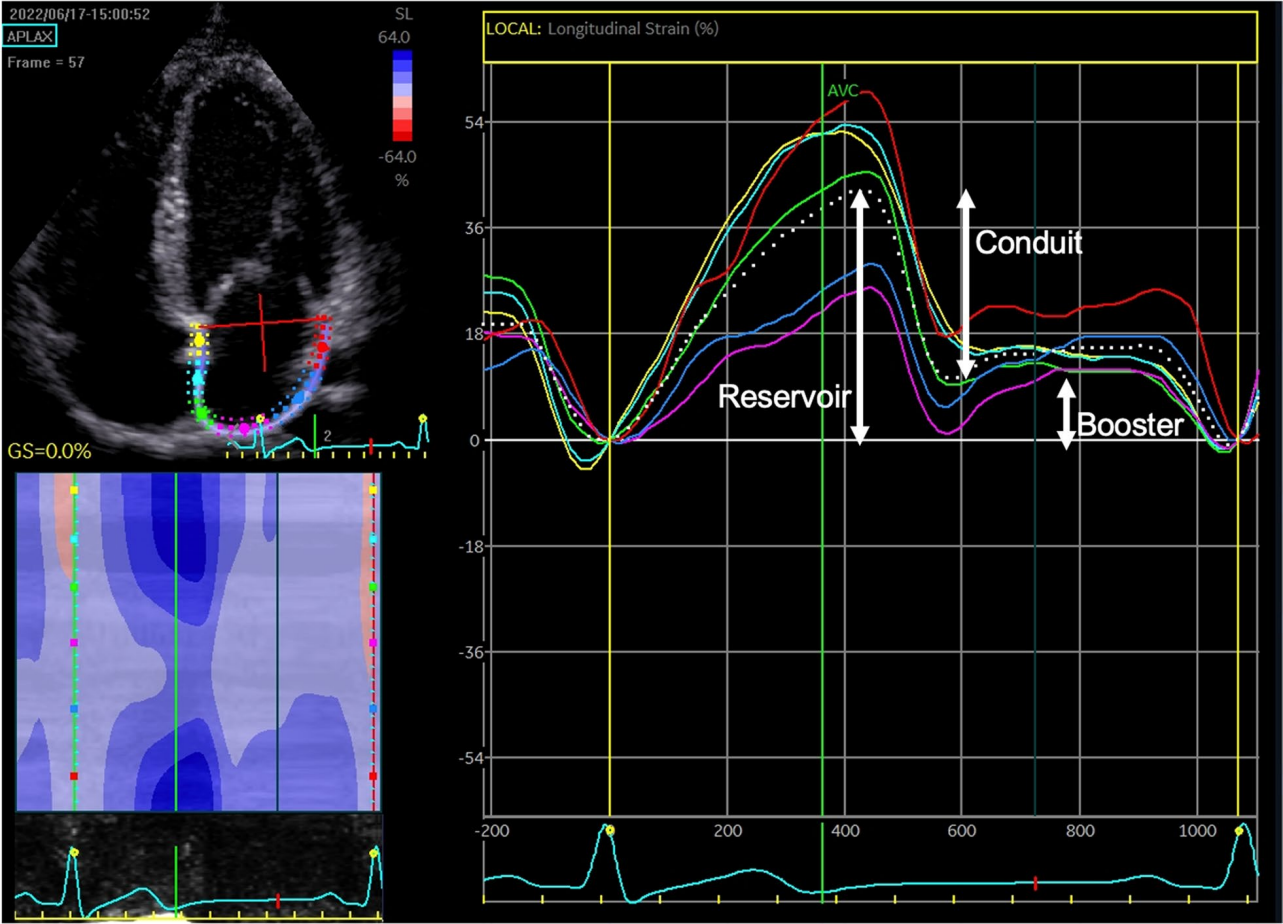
Measurement of left atrial reservoir, conduit and booster strain in the apical four chamber view
